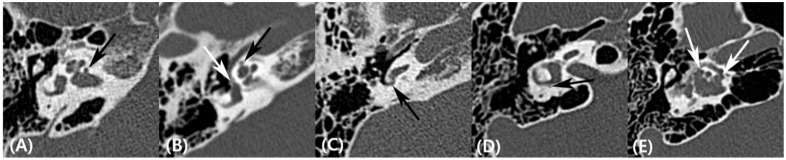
(A) Axial computed tomography (CT) image showing the presence of a focal hypodense notch connecting to the anterior wall of the internal auditory canal (IAC), designated as a cavitating lesion with IAC involvement (arrow). (B) Axial CT image showing otosclerotic involvement of the cochlear (black arrow) and the vestibule (white arrow). (C,D) Axial CT images showing retrofenestral involvement of the (C) round window and (D) the lateral semicircular canal (arrows). (E) Confluence between the cavitating lesion involving the IAC and cochlear involvement was demonstrated on axial CT (arrows).
